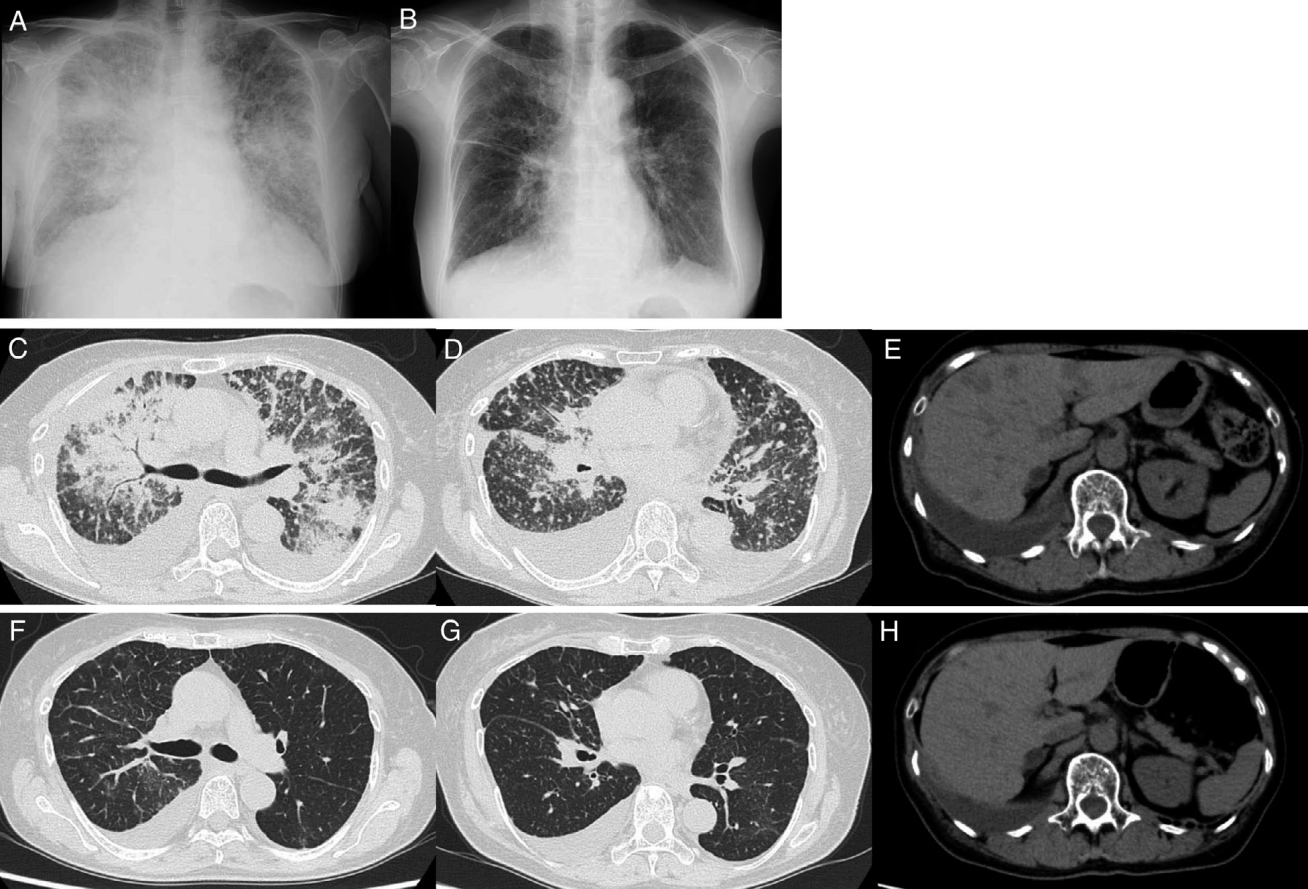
Images showing the patient's response to dabrafenib and trametinib treatment. (A, B) Radiographs of the chest, (A) before the treatment on day 1 and (B) after the treatment on day 15. (C, D, F, G) Computed tomography (CT) of the chest and lung window settings and (E, H) CT of the abdomen and mediastinal window settings. (C–E) Before the treatment on day 1 and (F–H) after the treatment on day 31
